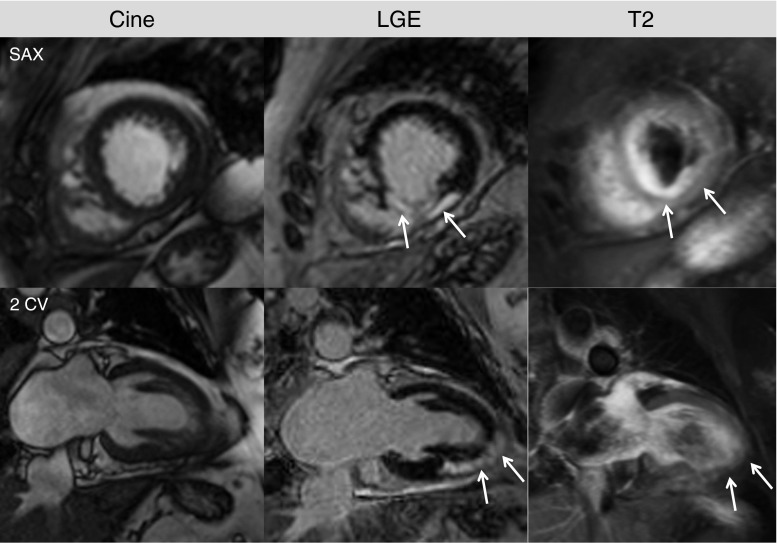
A 61-year-old female presenting with chest pain and elevated cardiac enzymes. Coronary artery disease (CAD) was ruled out by coronary angiography. a Cine images showed a slightly diminished left ventricular ejection fraction (LVEF = 53 %). b LGE images revealed an almost transmural LGE inferoseptal from midventricular to the apex, from epicardial origin. This pattern could indicate cardiac manifestation of a systemic disease such as sarcoid. c T2-weighted images demonstrate hyperintense regions (edema) in the inferoseptal wall (*white arrows*), corresponding to LGE. Biopsies were taken, which confirmed the diagnosis of sarcoidosis, and steroid therapy was initiated
